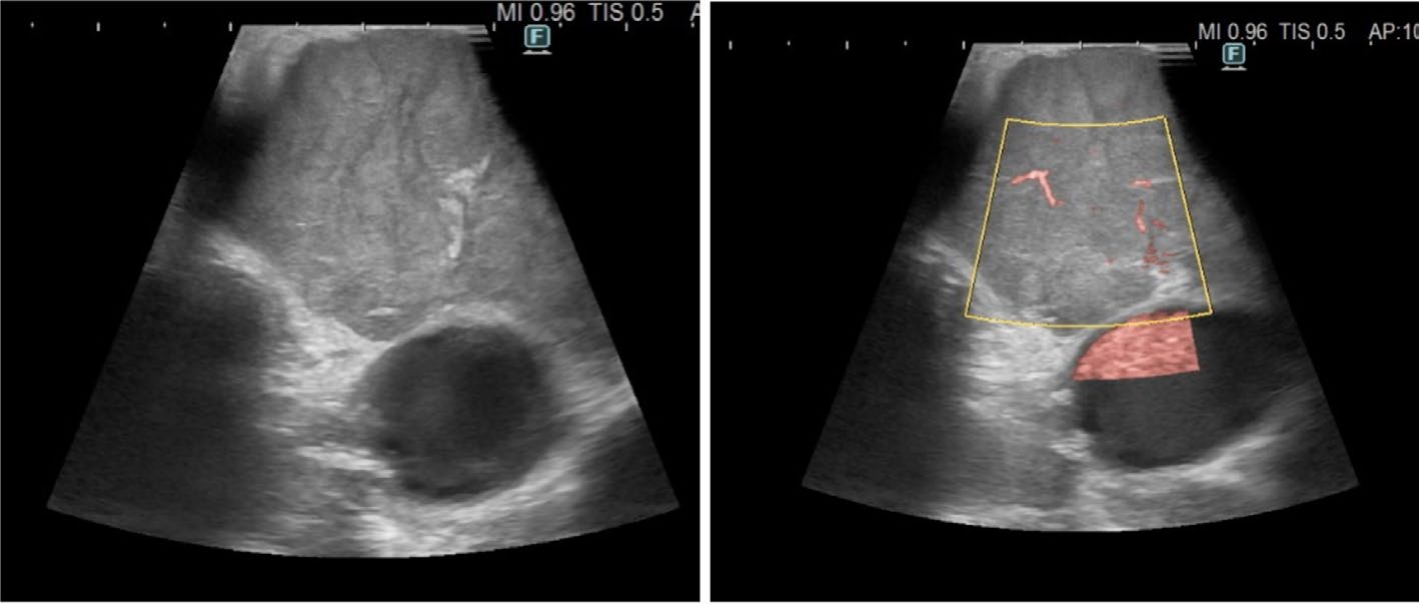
AMMs were classified based on their ultrasound characteristics, including shape, echogenicity, short‐axis diameter, margin, increased color Doppler flow, presence or absence of coagulative necrosis, presence or absence of calcification, nodal conglomeration, and vascularization of the lesion.
